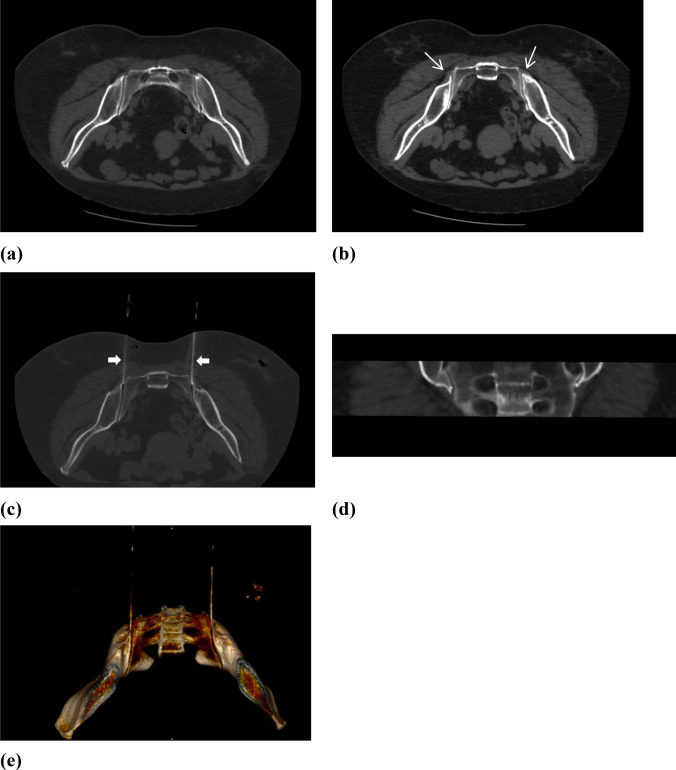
CT-guided SIJ injection in a 27-year-old female with bilateral inflammatory sacroiliitis. No degenerative changes are noted. Axial images show capsule distension (a and b), needle placement within the inferior joint compartments (c), and confirmation via a coronal image (d) and 3D reconstruction (e)
